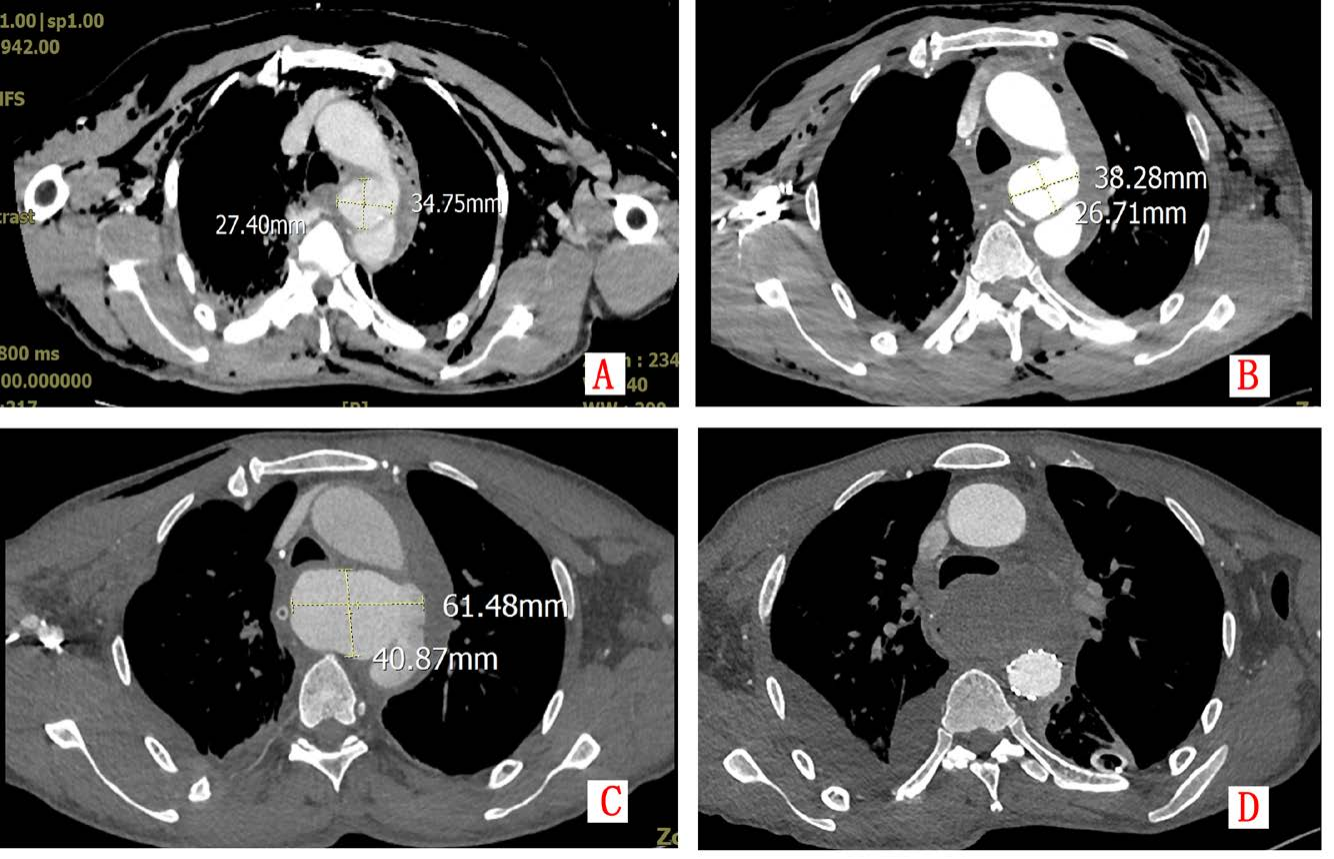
(A–C) Contrast-enhanced CT demonstrates progressive enlargement of a pseudoaneurysm; (D) post-stent implantation imaging reveals stable positioning without contrast leakage.
